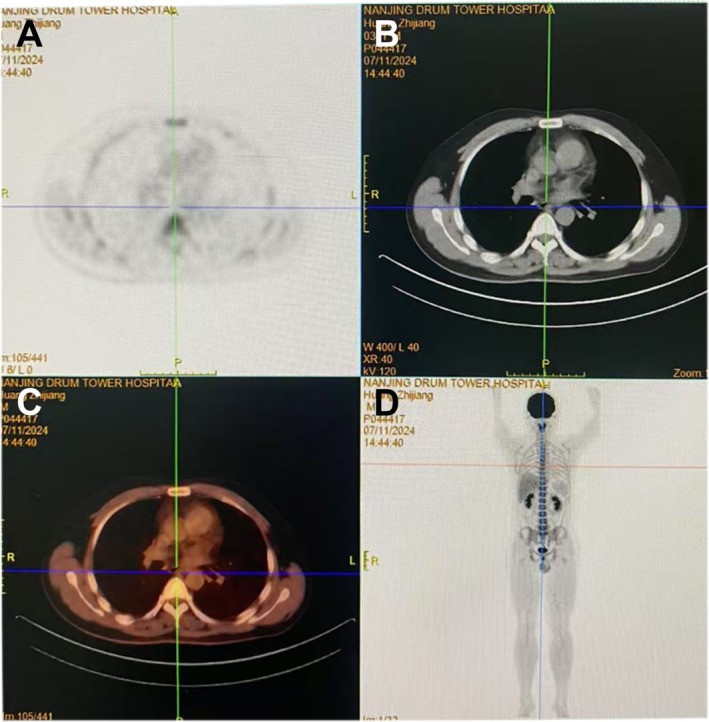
Positron‐emission tomography/computed tomography images of the patient. (A, B) Cross‐sectional computed tomography (CT) image of the esophagus area. (C) Cross‐sectional functional imaging images of the esophagus area. (D) Coronal functional imaging images of the esophagus area.
